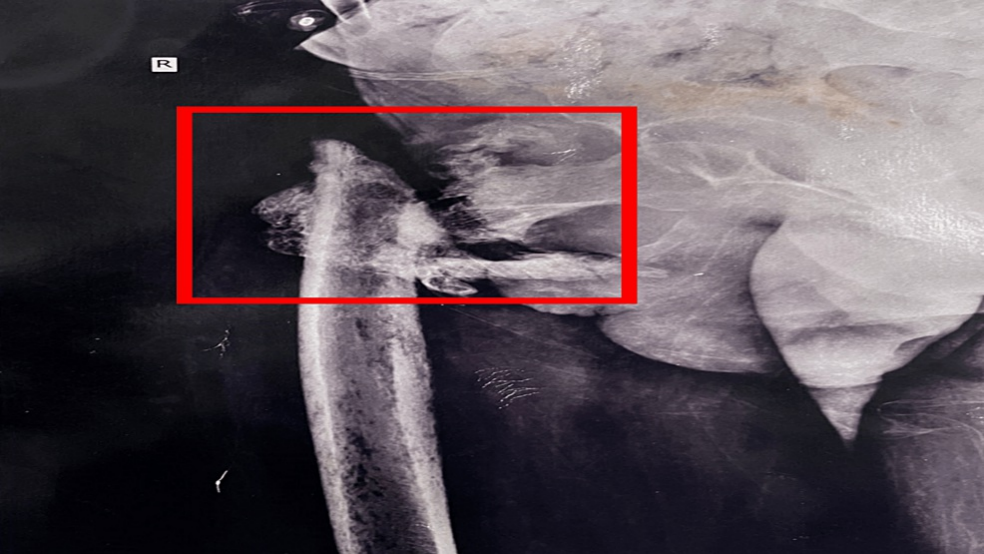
Preoperative X-ray of the anteroposterior view of the right hip
